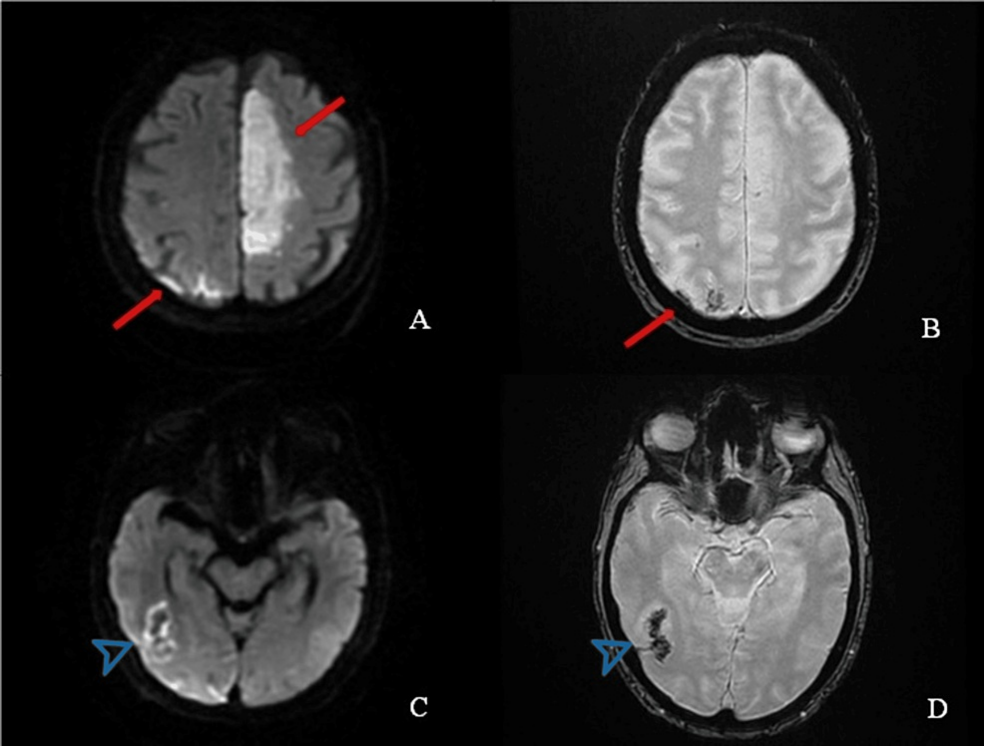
A brain MRI of the first patient with HBC-3 hemorrhagic conversion following tenecteplase administration Axial diffusion-weighted imaging (DWI) (A) and gradient recalled echo (GRE) (B) sequence MRI images of the brain demonstrate diffusion restriction in the left anterior cerebral artery (ACA) territory and right para-midline parietal lobe compatible with bilateral acute-subacute infarcts (red arrows). Axial DWI (C) and GRE (D) images of the brain at the level of the parieto-occipital lobe demonstrate diffusion restriction and susceptibility artifacts in the right para-midline parietal and occipital lobes compatible with intraparenchymal hemorrhage (arrowhead). HBC: Heidelberg bleeding classification
